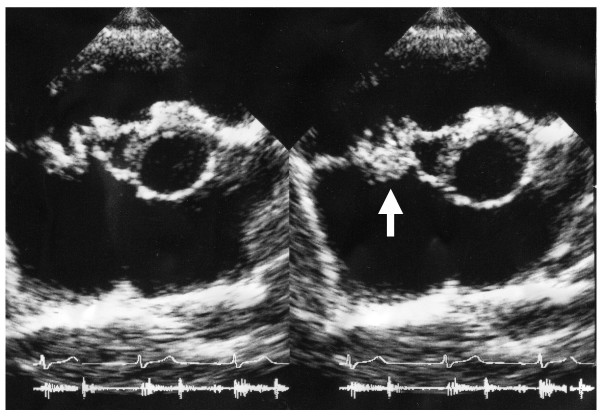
Vegetation on tricuspid valve by echocardiography. Arrow denotes the vegetation.
